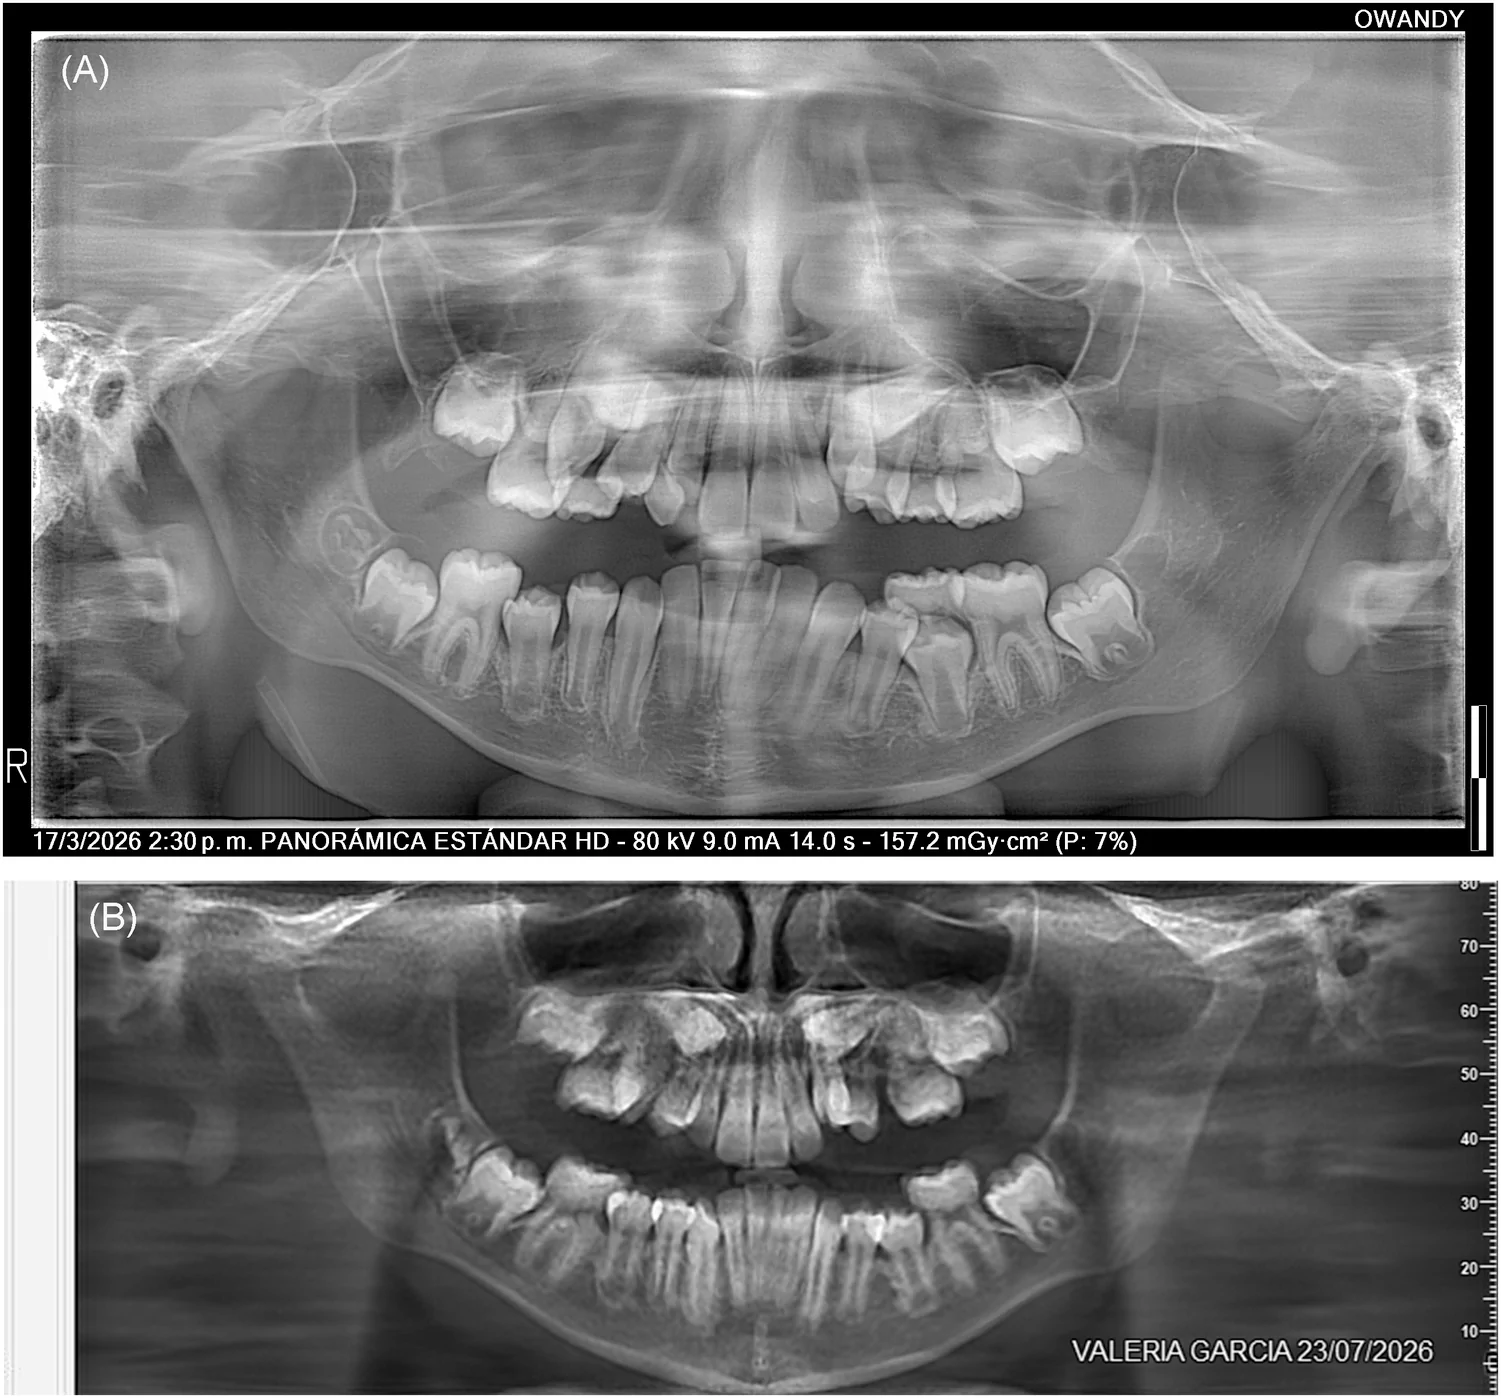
Radiographic evaluation during longitudinal follow-up. (A) Initial panoramic radiograph obtained before comprehensive dental treatment, demonstrating the mixed dentition stage and treatment needs. (B) Follow-up panoramic radiograph obtained six months after treatment, showing satisfactory healing following dental extractions, normal eruption of the permanent dentition, and the absence of postoperative complications.
